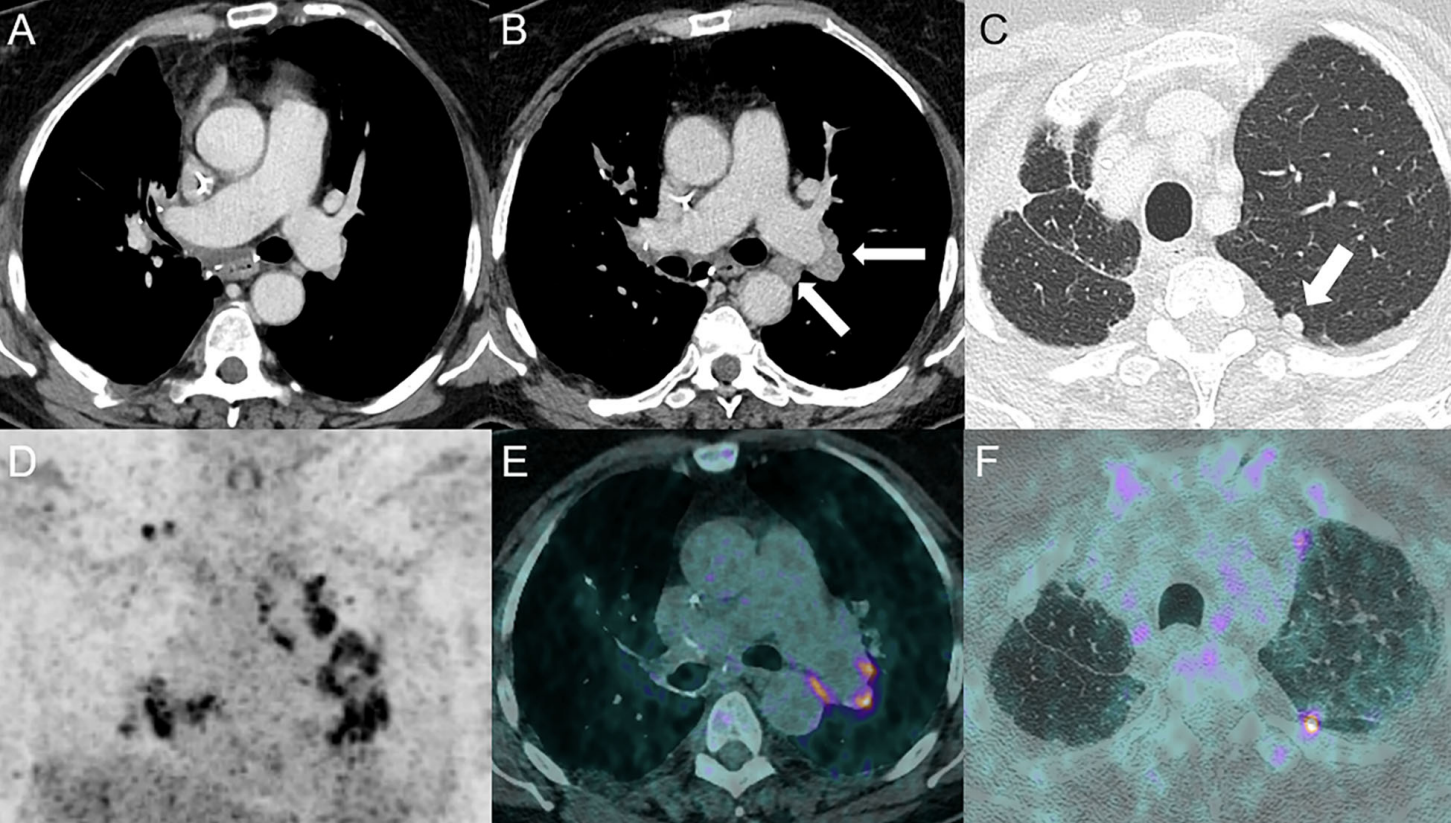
Sarcoidosis-like reaction in a 69-year-old female with stage IIIa lung adenocarcinoma in the right upper lobe treated by neoadjuvant cisplatin-docetaxel followed by durvalumab (anti-PD-L1) with subsequent right upper lobe lobectomy and lymphadenectomy. The patient received adjuvant durvalumab 1 month post-surgery. Baseline CT following surgery is shown in (A). Follow-up CT at 5 months showed the development of bilateral hilar and mediastinal lymphadenopathies [arrows, (B)] and an increasing nodule in the left upper lobe (arrow) (C). ^18^F-FDG PET-CT confirmed high uptake of mediastinal and hilar lymph nodes [PET (D) and fused PET/CT (E) images] and the upper left lobe nodule [fused PET/CT images (F)]. A wedge resection confirmed the sarcoidosis-like nature of the nodule.
